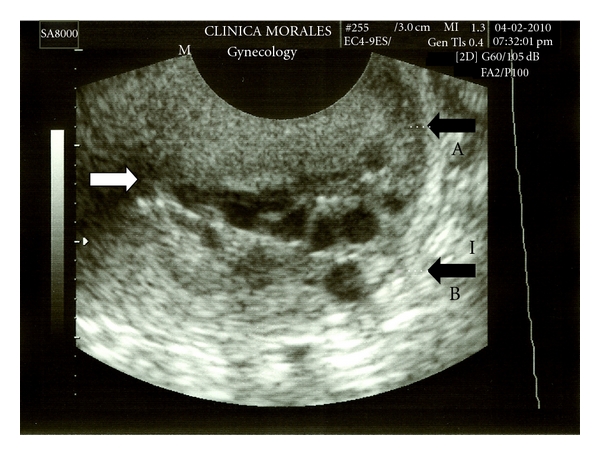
Transvaginal ultrasound depicts an axial section of the uterus; a normal anteroflexed uterus is seen with a double cervix (black arrows A, B). Both endocervical channels join together. White arrow points to the place at the internal cervical os where the channels fuse.
